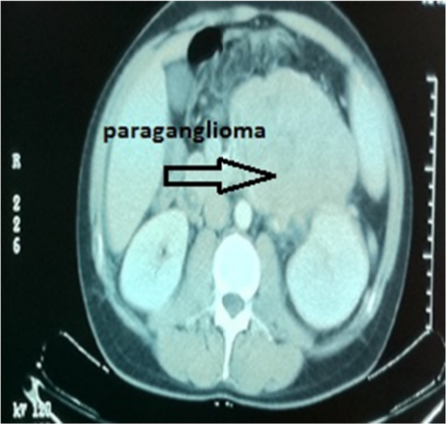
Pre-operative CT image of malign paraganglioma.
